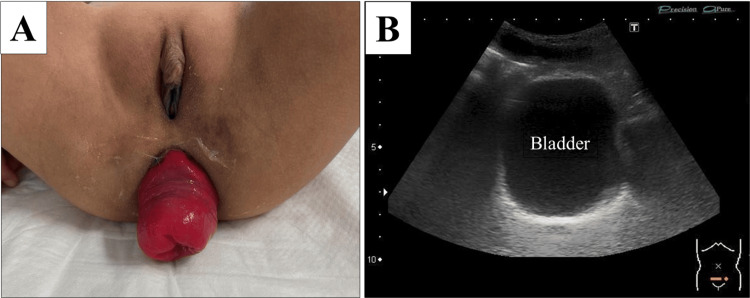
Physical and ultrasonographic findings. (A) Rectal prolapse in the outpatient clinic. (B) Ultrasound of the bladder showing a 5-mm-thickened calcification of the entire bladder wall.
